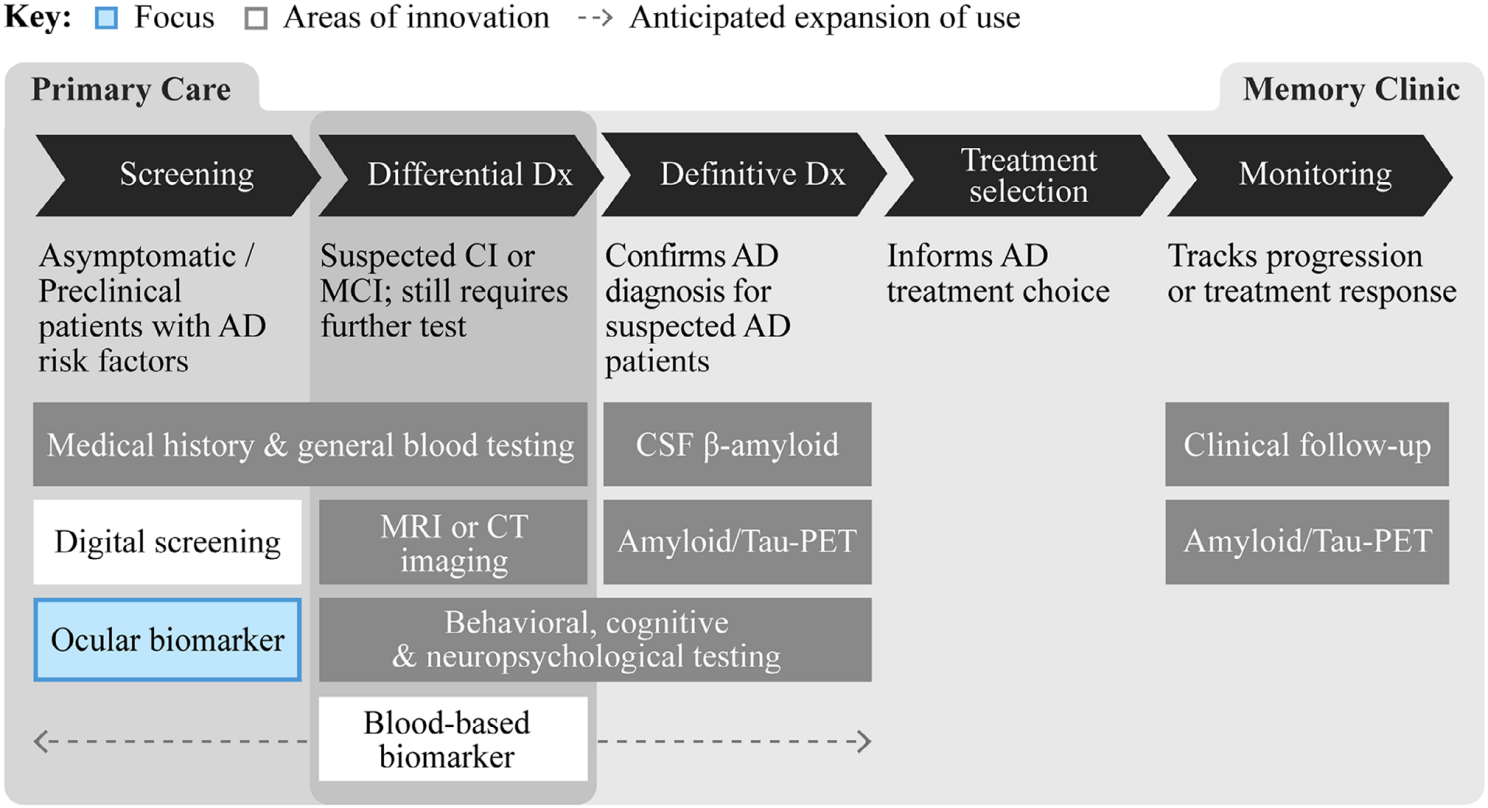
Potential future diagnostic paradigm for AD. In the current diagnostic paradigm for AD, digital tools are emerging for screening use cases, while blood‐based biomarkers are soon to become critical tools in differential diagnosis. Ocular technologies hold the most promise for the screening use case, to be used in tandem or potentially in place of digital screening tools. AD, Alzheimer's disease; CI, cognitive impairment; CSF, cerebrospinal fluid; CT, computed tomography; Dx, diagnosis; MCI, mild cognitive impairment; MRI, magnetic resonance tomography; PET, positron emission tomography.
